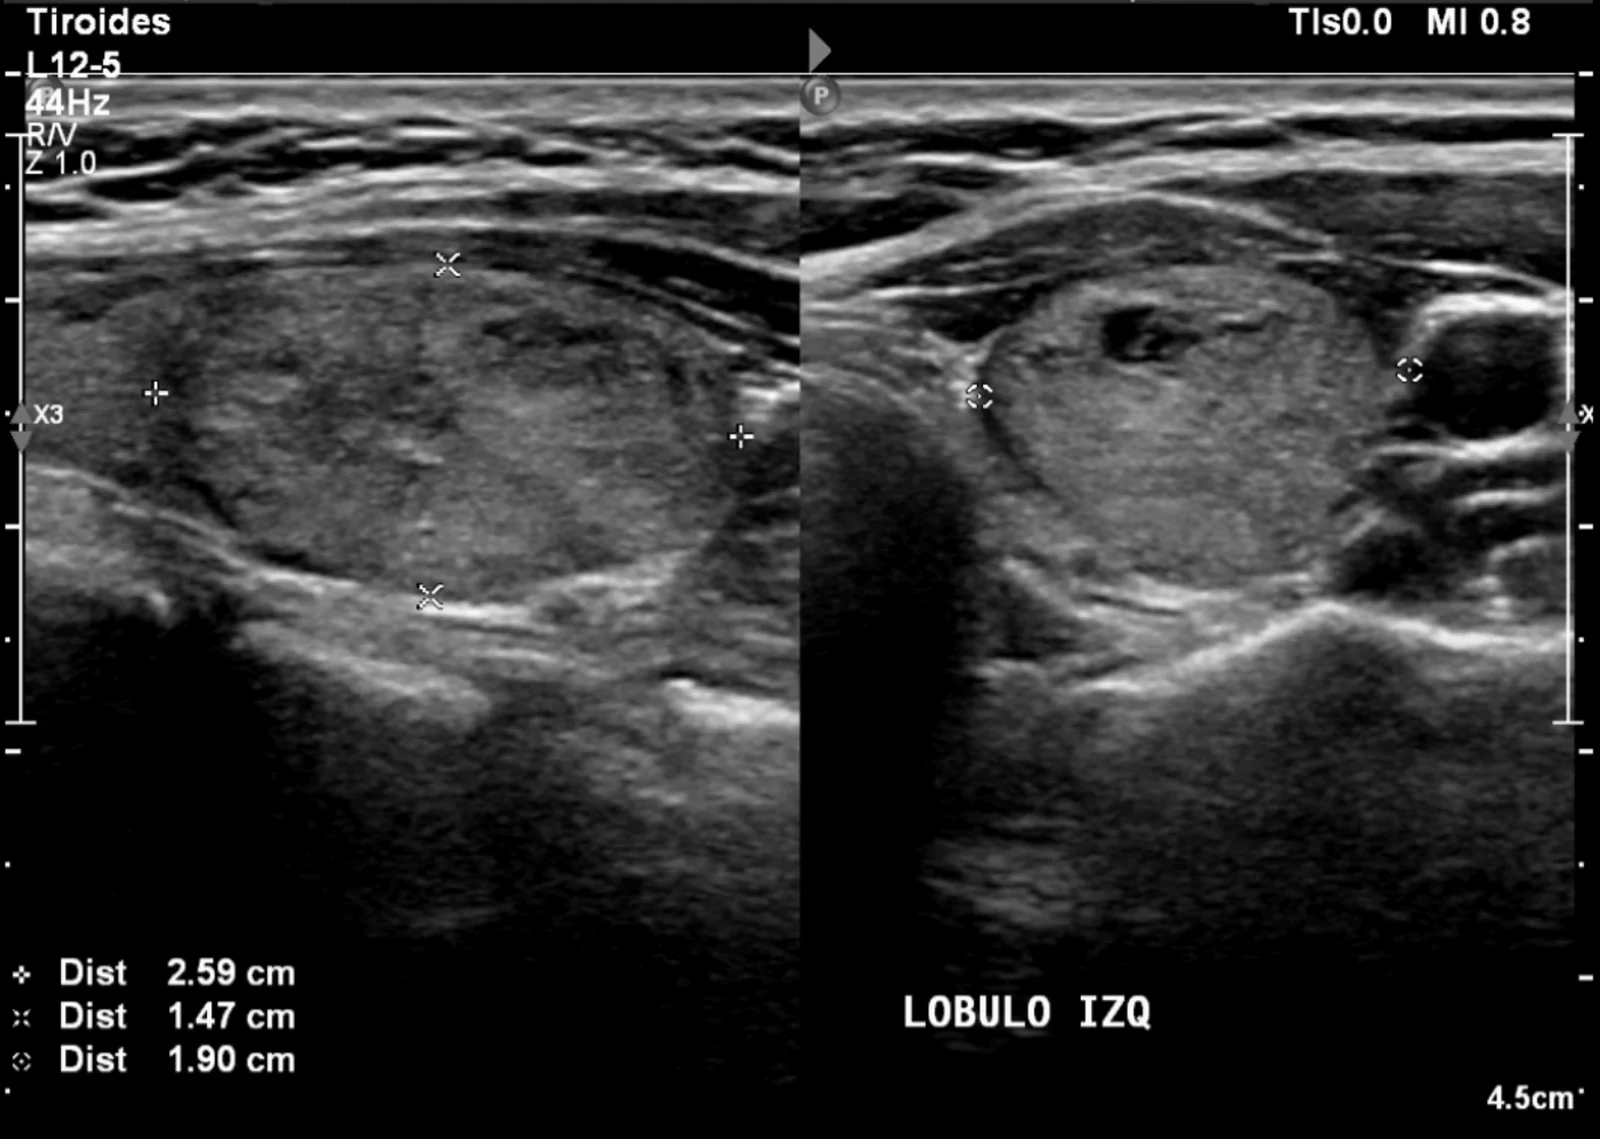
Thyroid ultrasound showing a TI-RADS 4A nodule in the left thyroid lobe Thyroid ultrasound (LOBULO IZQ) demonstrating a heterogeneous solid nodule with a hypoechoic halo, mixed solid-cystic components, and circumscribed margins, measuring 2.59 × 1.47 × 1.90 cm, located at the junction of the middle and lower thirds of the left thyroid lobe. The nodule was classified as TI-RADS 4A. Fine-needle aspiration biopsy returned Bethesda II; however, serum thyroglobulin was elevated at 30 ng/mL, prompting surgical resection. Definitive pathology identified a papillary thyroid carcinoma, encapsulated follicular variant (pT2). TI-RADS: Thyroid Imaging Reporting and Data System
